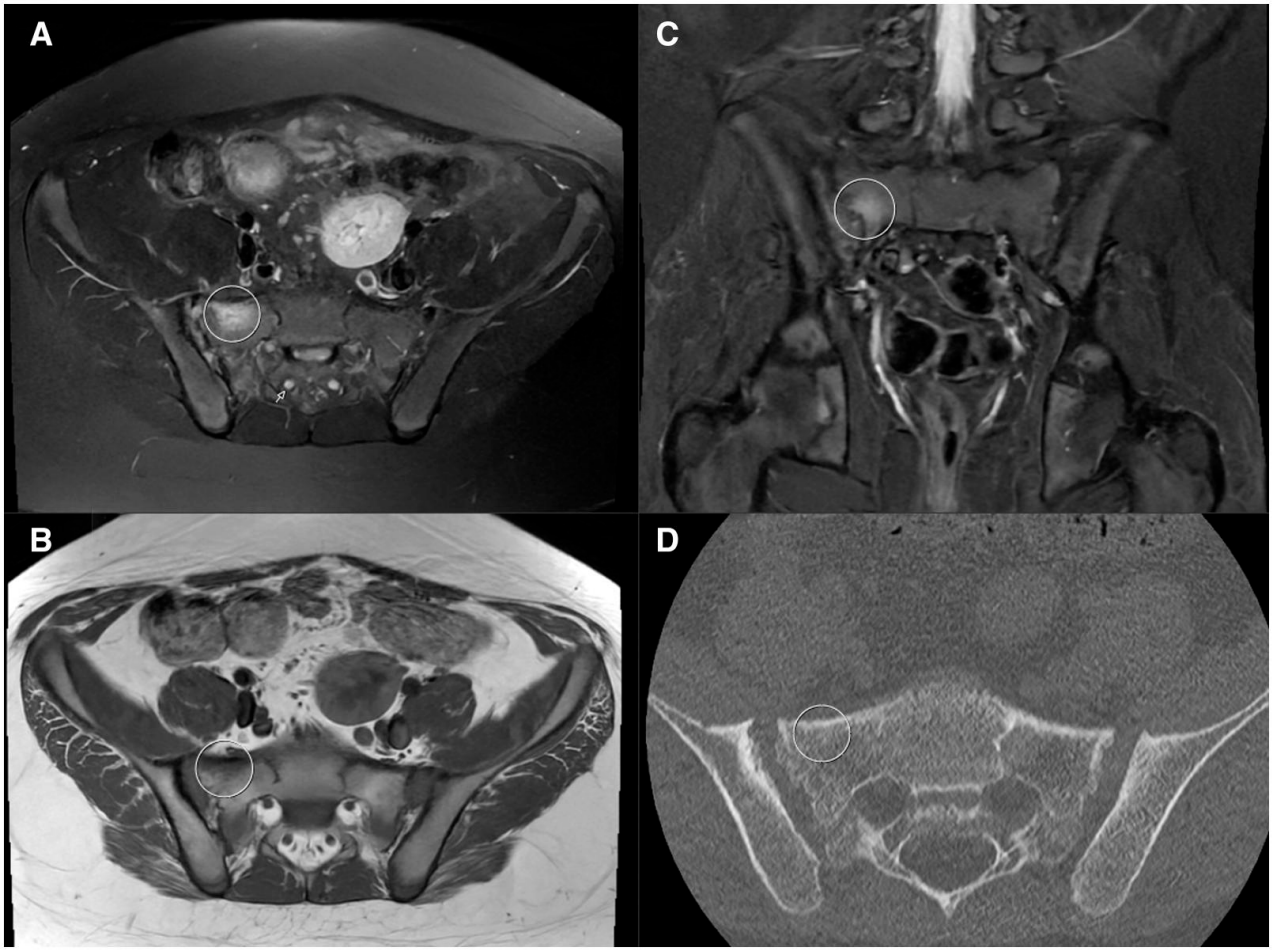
Compatible with the intense oedema area on the right sacral face, an increase in signal (circle) is observed in the axial T2-weighted fat-suppressed MRI section (A), a decrease in signal (circle) in the axial T1-weighted non-contrast MRI section (B), and an increase in signal (circle) in the coronal STIR section (C). Note that in both the T2 fat-suppressed and STIR MRI scans, the signal intensity is similar to the cerebrospinal fluid (CSF) signal in the sacral neural foramen (white arrow). (D) Axial image of ldCT.
